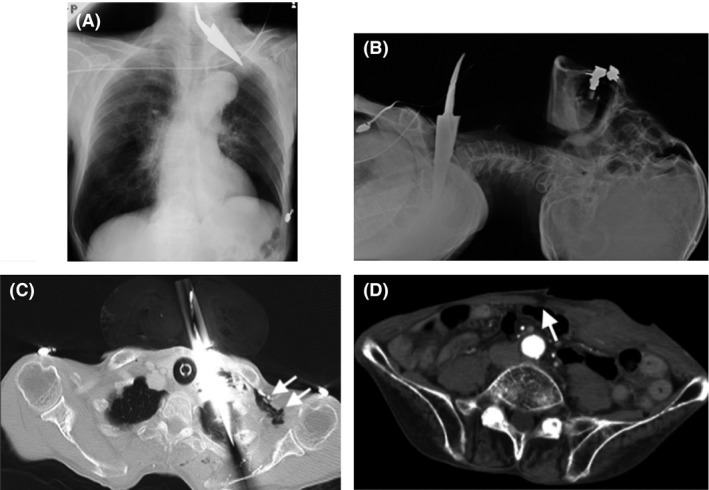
Radiographs and whole body computed tomography (CT) scans taken on admission of an 84‐year‐old man with self‐inflicted stab wounds to the neck and abdomen. A, Radiograph of the chest. B, Radiograph of the neck. C, CT image of the chest. Arrows indicate the air leakage. D, CT image of the abdomen. The arrow indicates the injury to the posterior layer of the rectus sheath.
